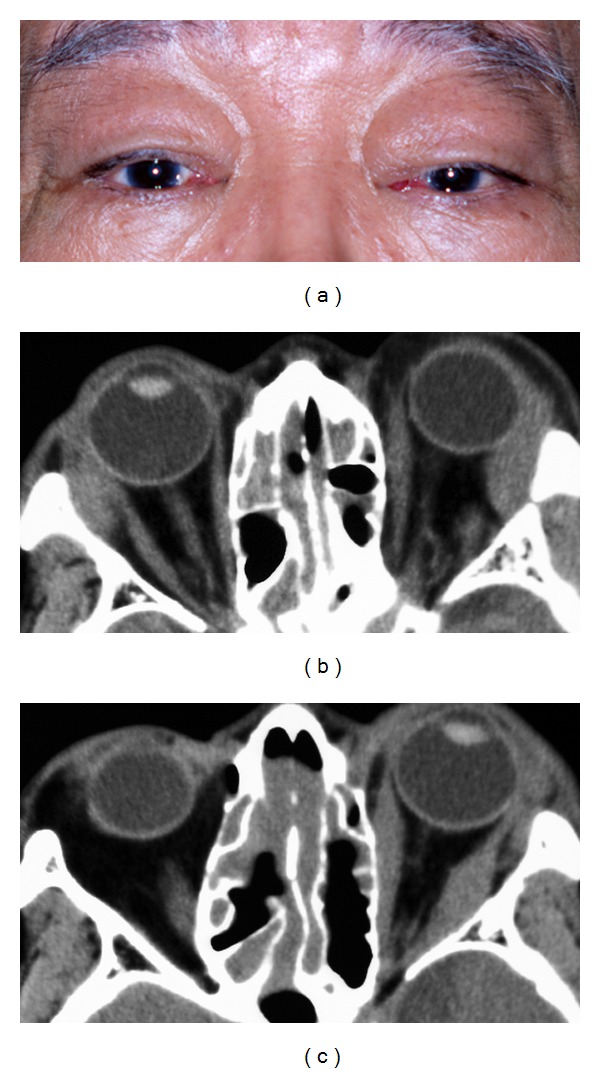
A typical case of orbital IgG4-related disease. A 72-year-old man with bilateral upper eyelid swellings that developed over a one-year period (a). There was a 10-year history of chronic rhinosinusitis, and the patients had undergone surgical treatments. On examination, his best-corrected visual acuity was 20/20 OD and 20/25 OS. Computed tomography showed enlargements of both lacrimal glands (b), enlargement of several of the left extraocular muscles (c), and infiltrative lesions in the ethmoid and maxillary sinuses. However, his ocular movements were unrestricted, and diplopia was not observed. He had elevated levels of serum IgG (4205 mg/dL; normal range: 870–1700 mg/dL) and serum IgG4 (1190 mg/dL; normal range: 4.8–105 mg/dL). These infiltrative lesions decreased in size after administration of oral prednisolone (30 mg) with a slow taper.
